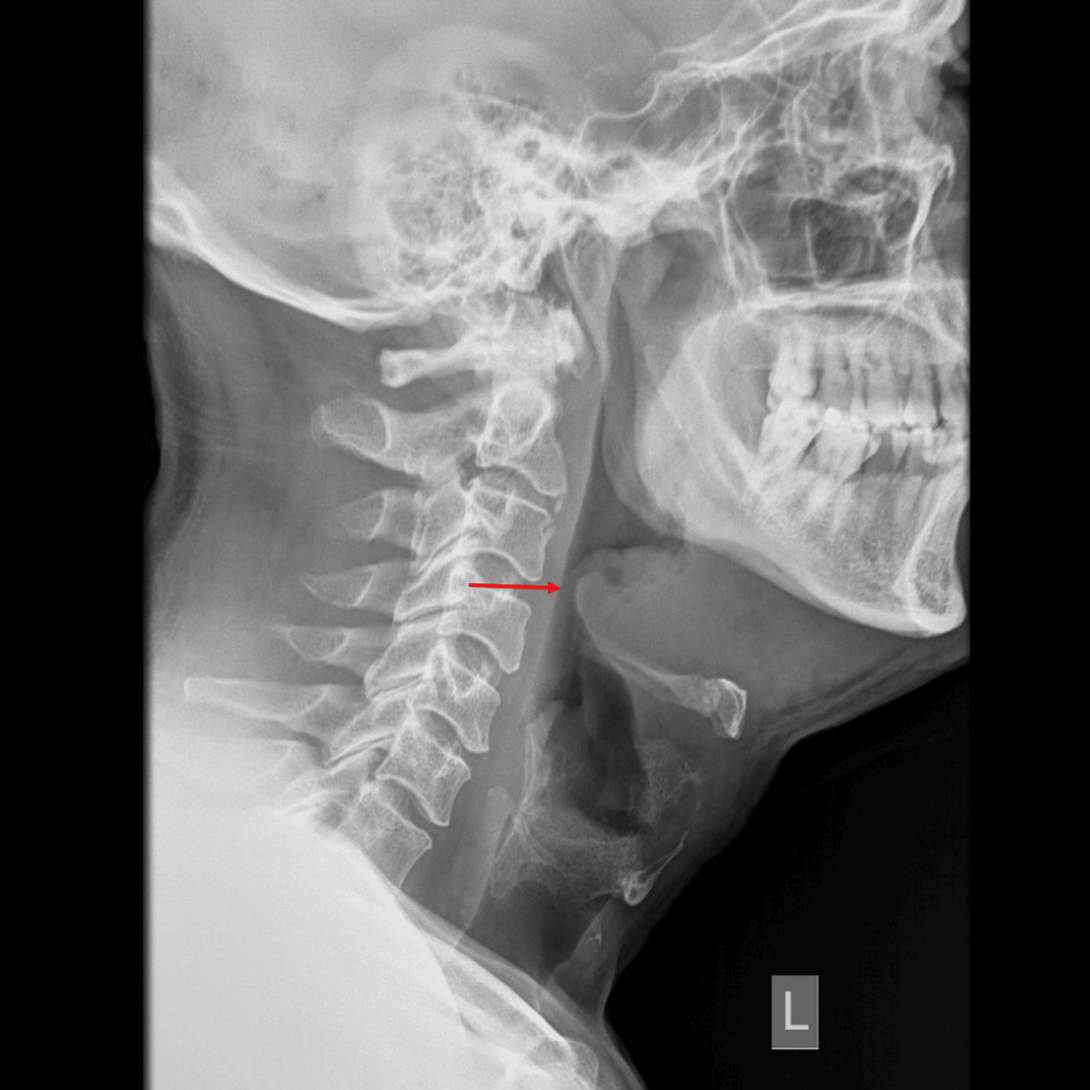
Soft tissue neck X-ray showing the enlarged epiglottis (thumbprint sign)
